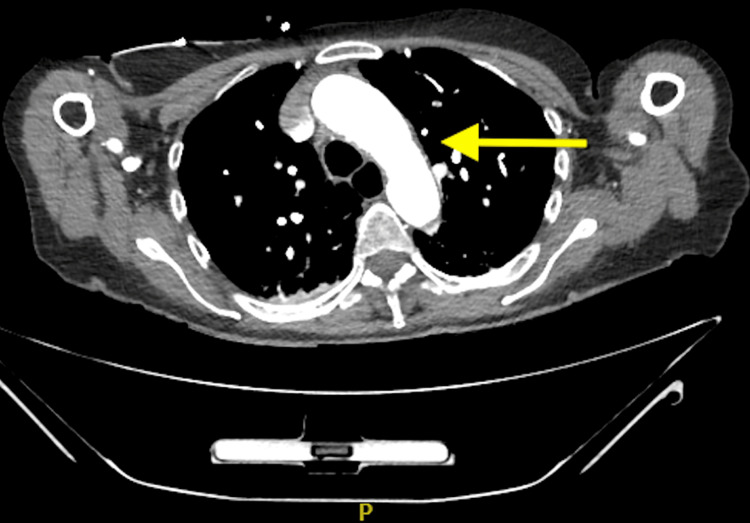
An axial, contrast-enhanced CT image of the thoracic aorta revealed no evidence of aortic dissection and aneurysm.
